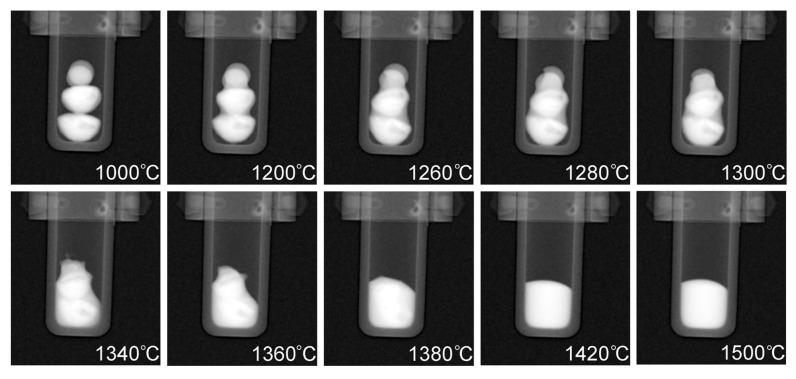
Radiographs taken at increasing temperatures of the CoCrCu + Ni buttons inside the alumina crucible in the HOT-A vacuum furnace. A full movie-like sequence of radiographs can be found in the Supplementary Materials.
